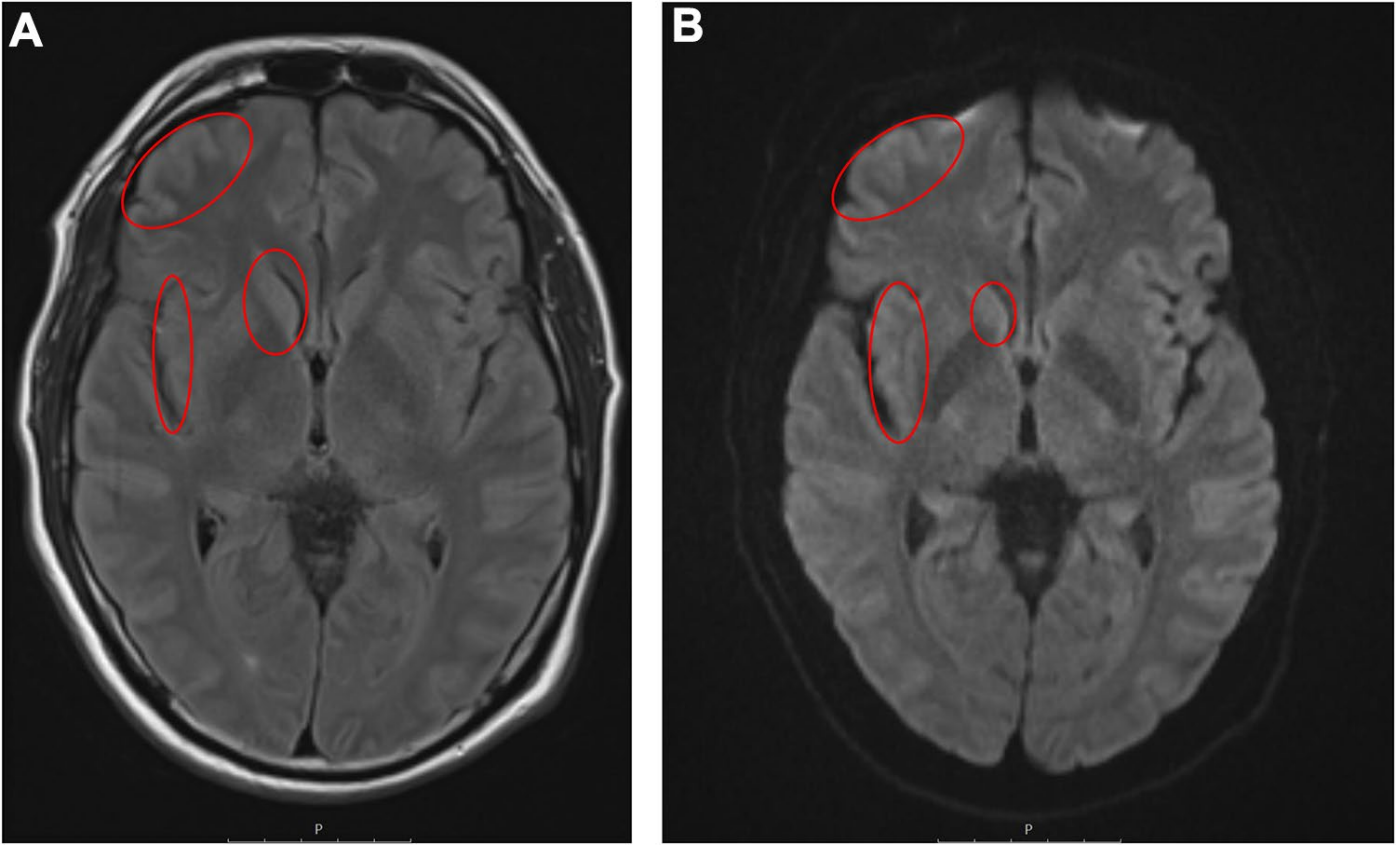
Cerebral MRI obtained on day seven after hospitalisation. The axial fluid-attenuated inversion recovery (FLAIR) sequence shows symmetric elevation in the caudate nuclei, the insular ribbon and the cortex A. No contrast media enhancement was visible. An axial diffusion-weighted imaging (DWI) sequence with a corresponding signal elevation (without signal attenuation in apparent diffusion coefficient (ADC) mapping, not shown) is depicted in B. For better clarity of the image, symmetric signal elevations were only marked on one side
